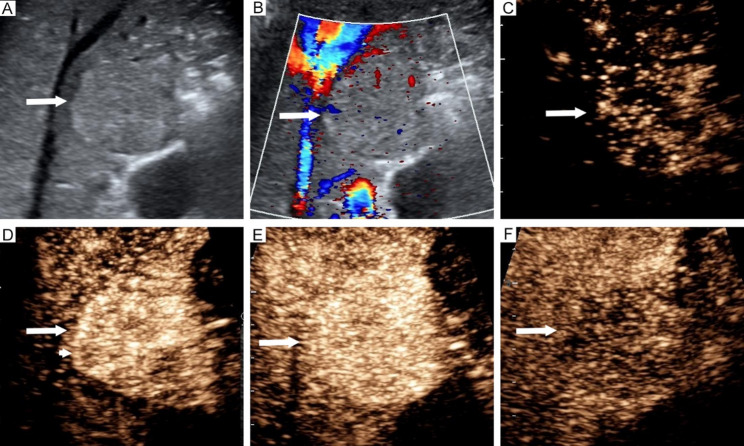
Imaging features of glypican-3-negative hepatocellular carcinoma in a 63-year-old man. Isoechoic lesion in segment 5 (a). Blood flow in the lesion (b). Contrast-enhanced ultrasound reveals early enhancement at 12s (c), hyper-enhanced rim (arrowhead) at 15s (d), and no necrosis at 21s, all within the arterial phase (e), followed by hypo-enhancement at 108s in the portal late phase (f). Arrow indicates the hepatocellular carcinoma lesion
